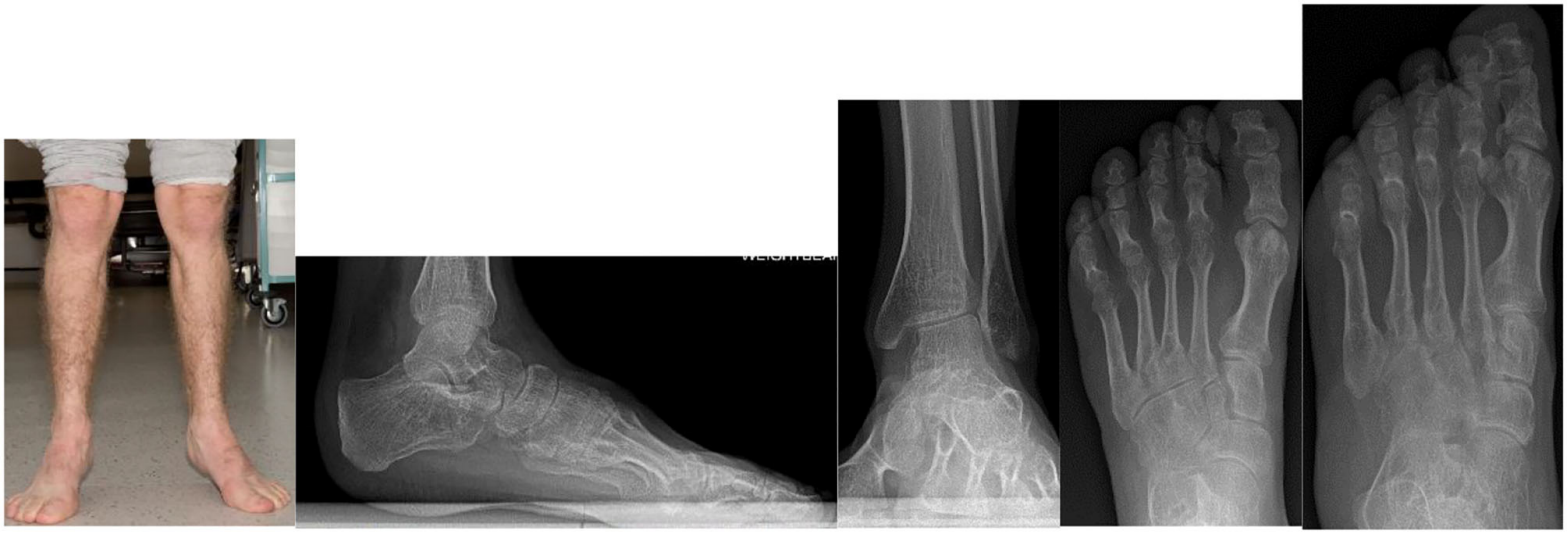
Clinical photograph of a 26- year- old male with MPS VI in fixed equinus contracture, caused by gastro-soleus spasticity secondary to spinal cord compression at the level of the conus medullaris. Radiographs of the left foot and ankle reveals distal tibial epiphyseal dysplasia with valgus wedging, decreased LDTA, syndesmotic widening with lateral talar shift and increased medial clear space suggestive of ligamentous deficiency, shortened “bullet” metatarsals and an hypoplastic intermediate cuneiform.
